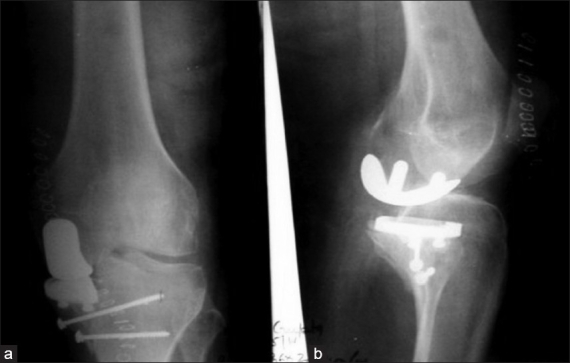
Immediate post-fixation anteroposterior view (a) and lateral view (b) radiographs of patient who had an undiagnosed medial tibial condyle fracture
